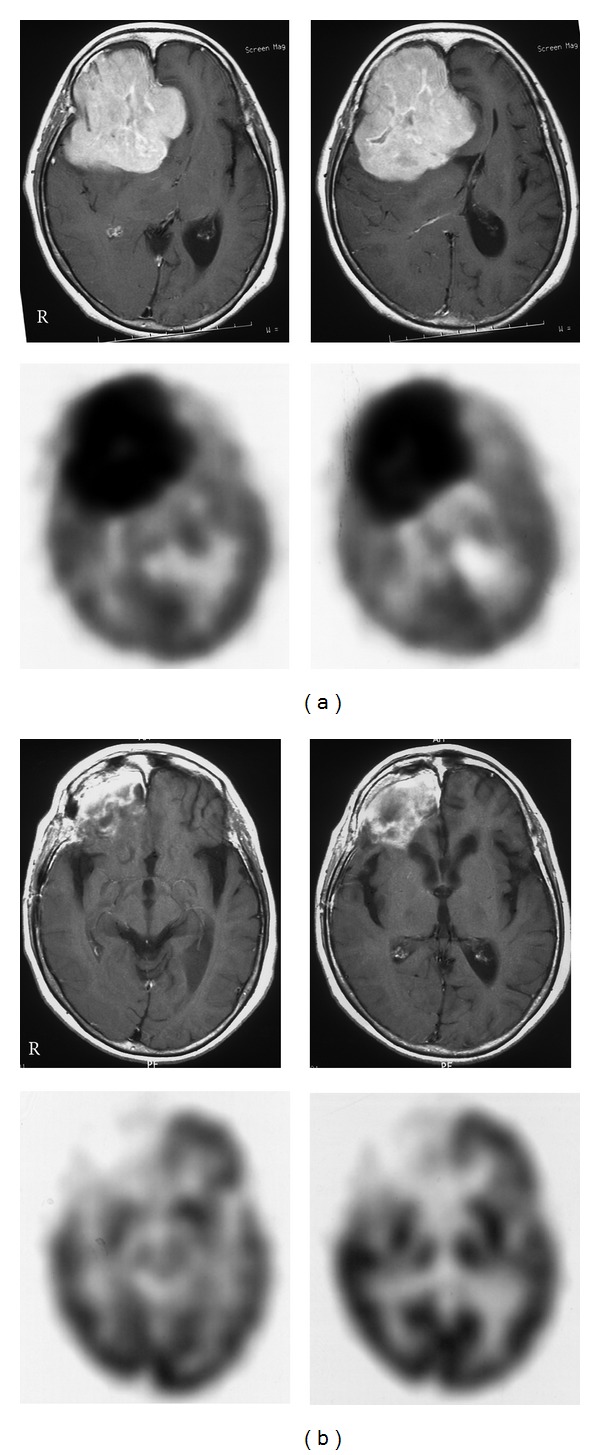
Contrast-enhanced T1-weighted MR (upper) and ^18^F-FDG PET (lower) images in a patient with PCNSL in the right frontal lobe before (a) and after (b) the first cycle of chemotherapy. MR images show a well-enhanced large mass lesion in the right frontal lobe, and PET images show a huge ^18^F-FDG uptake in the lesion before treatment (a). After the first chemotherapy, MR images show a residual enhanced lesion in the right frontal lobe; however, PET images show no increased ^18^F-FDG uptake in the lesion (b).
